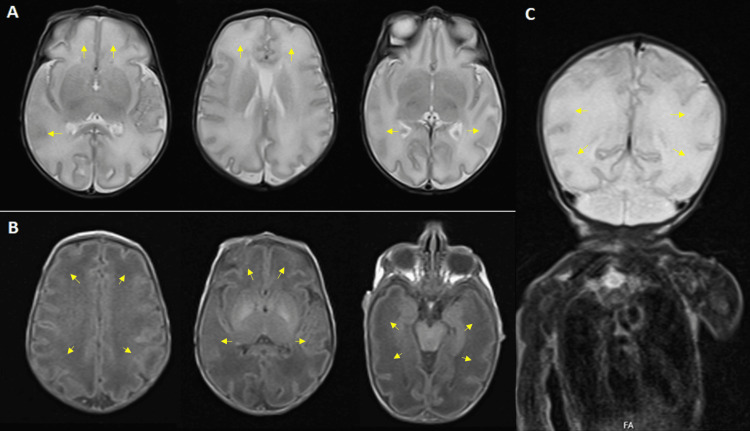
Brain MRI of twin B performed on the sixth day of life The scan revealed a simplified gyral pattern, primarily involving the frontal and temporal lobes (arrows), suggestive of a cortical developmental malformation. A: Axial T1-weighted view; B: Axial T2-weighted view; C: Coronal T1-weighted view.
